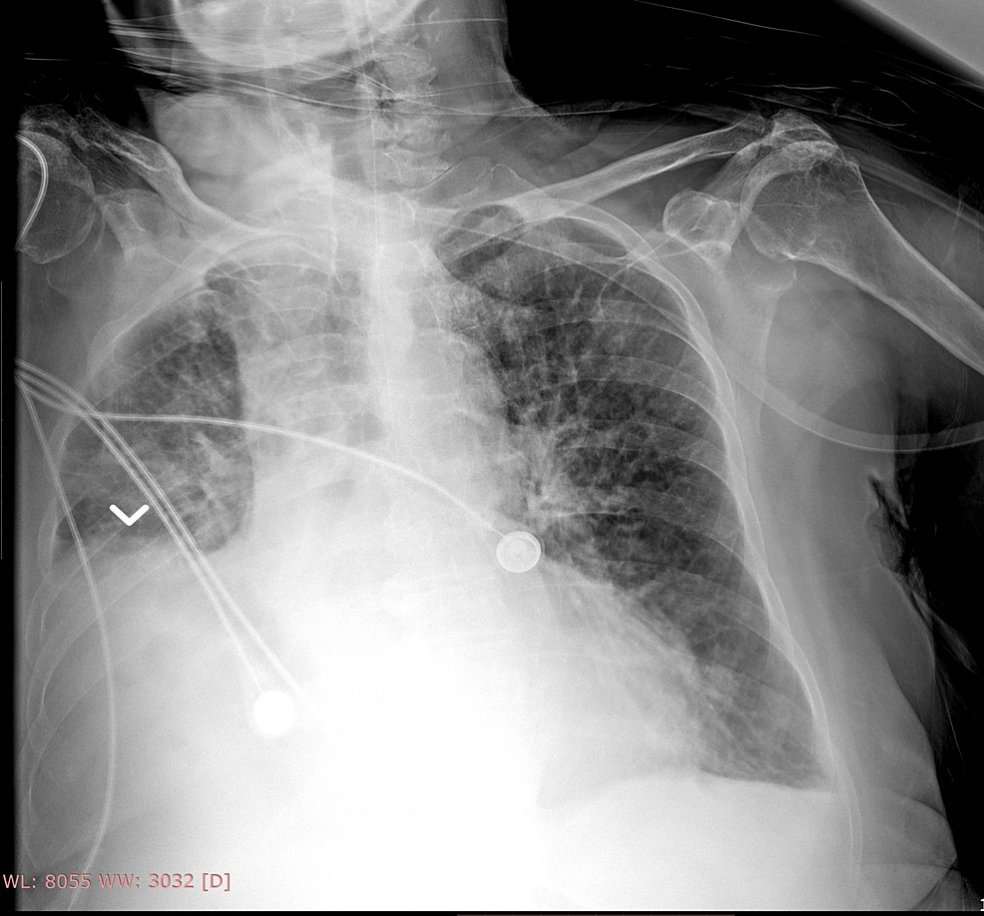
Portable chest X-ray at admission showing right lung lower lobe collapse (white arrowhead)
